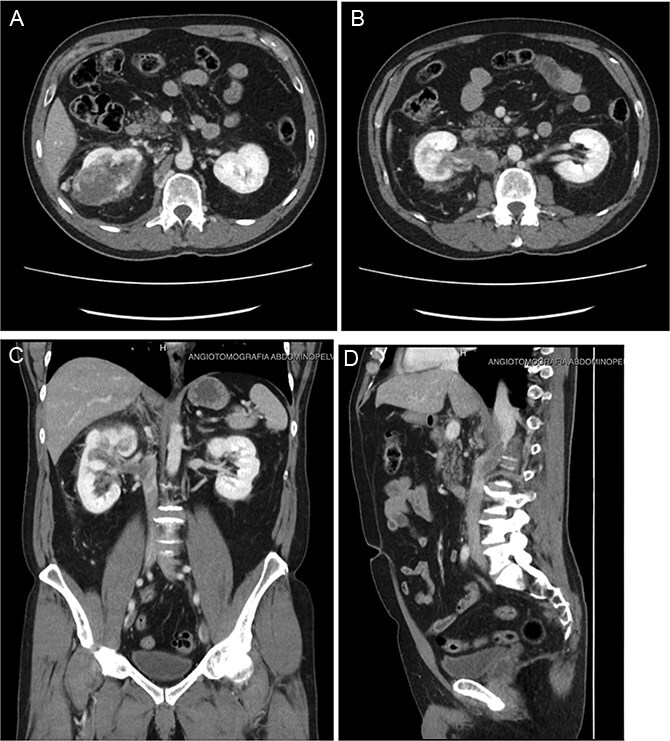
(A–D) Abdominopelvic contrast-enhanced computed tomography post-neoadjuvant therapy showed a significant reduction in tumor size and the involvement of the thrombus in the azygos vein.
